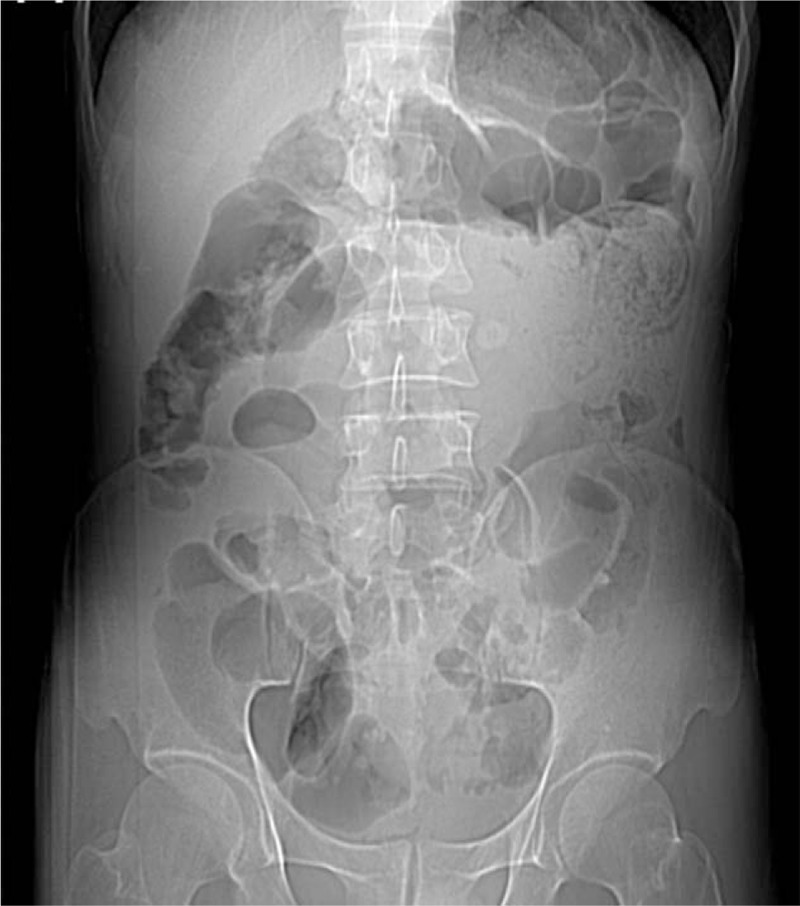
A contrast-enhanced CT of the abdomen showed a clear invagination of the small intestine.
